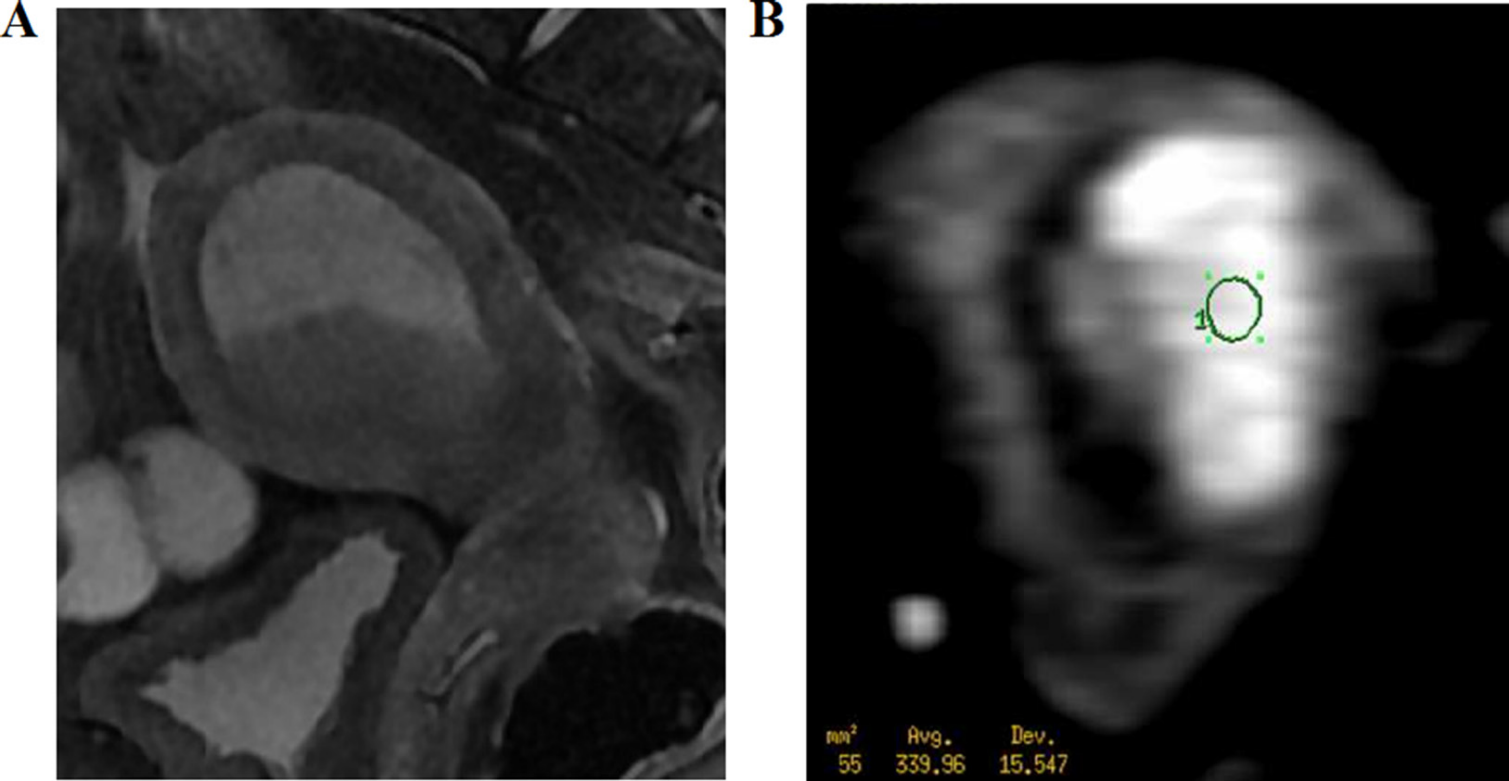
A 62-year-old woman with stage I endometrial carcinoma (A) Sagittal T2WI shows the carcinoma with slightly high intensity (arrow). (B) Axial DWI shows a carcinoma with hyperintensity. The region of interest (55 mm^2^) was placed at the center of the lesion.
